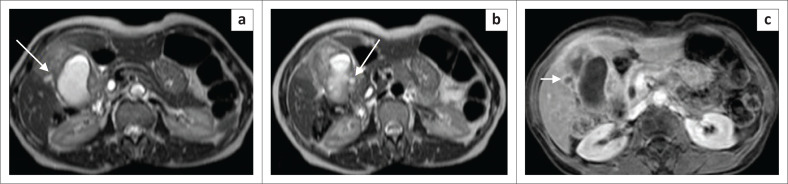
(a and b) Axial T2W magnetic resonance imaging images demonstrating hyperintense intramural nodules (arrows), which on post-contrast T1W sequence (c) shows peripheral enhancement (black arrow), suggestive of a microabscess.
